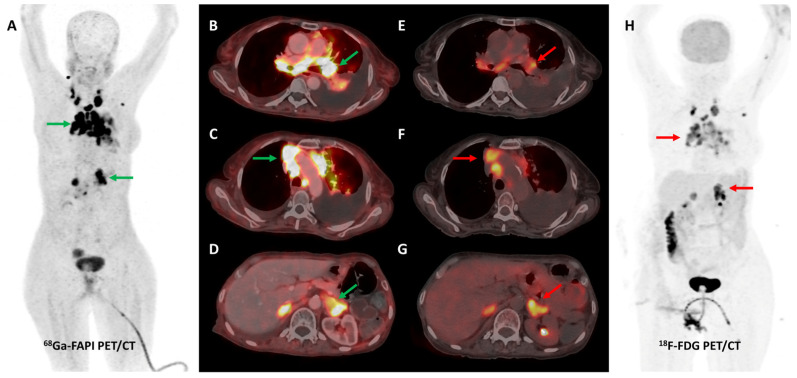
[^68^Ga]Ga-FAPI-04 and FDG PET/CT images in a 59-year-old woman with biopsy-proven metastatic left lung adenocarcinoma. [^68^Ga]Ga-FAPI-04 PET/CT images revealed intensely tracer avid left hilar mass lesion ((B)—green arrow), multiple enlarged mediastinal ((A,C)—green arrows) lymph nodes, and bilateral adrenal metastases ((A,D)—green arrows depicting left adrenal lesion). Additionally, moderate left-sided pleural effusion with associated left lung lower lobe collapse was noted. Overall, FAPI PET/CT demonstrated higher tracer avidity and TBRs than FDG PET/CT ((E–H)—red arrows).
